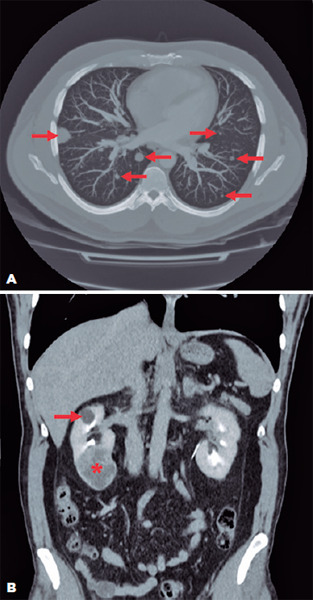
(A) Chest computed tomography (CT) image showing multiple nodules with a
